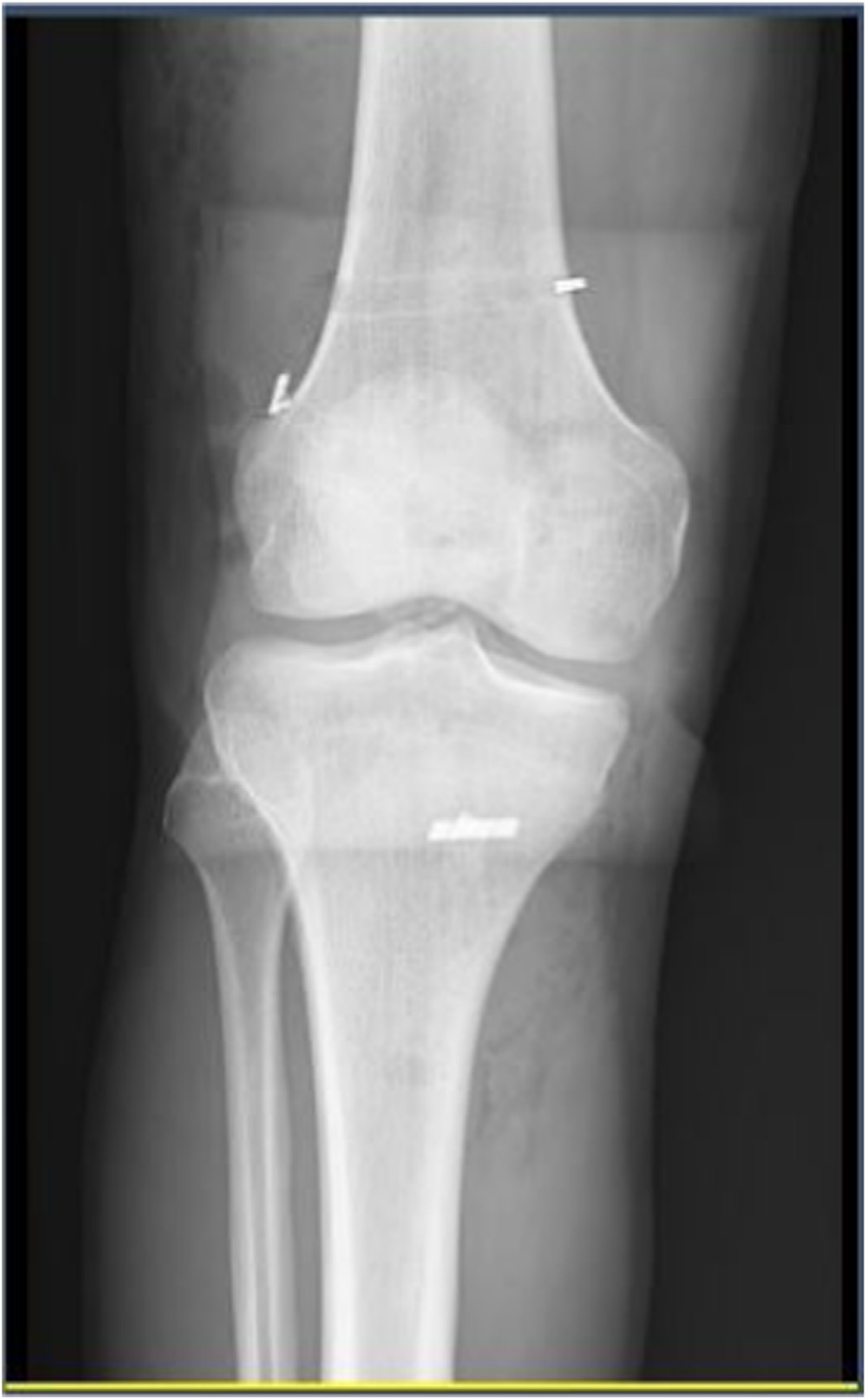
Postoperation knee radiography.
